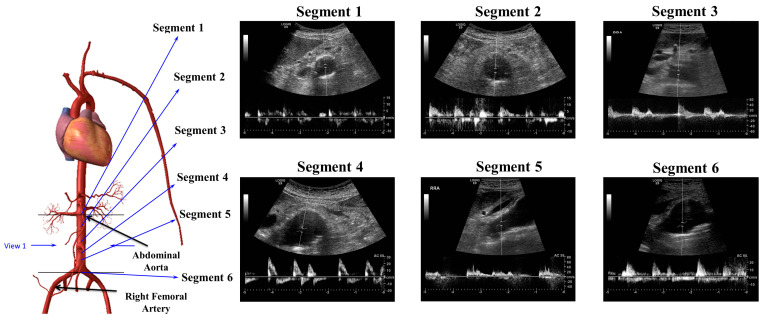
Stylized model of abdominal aorta and ultrasound data at different segments including circumference and flow velocities for each segment.
